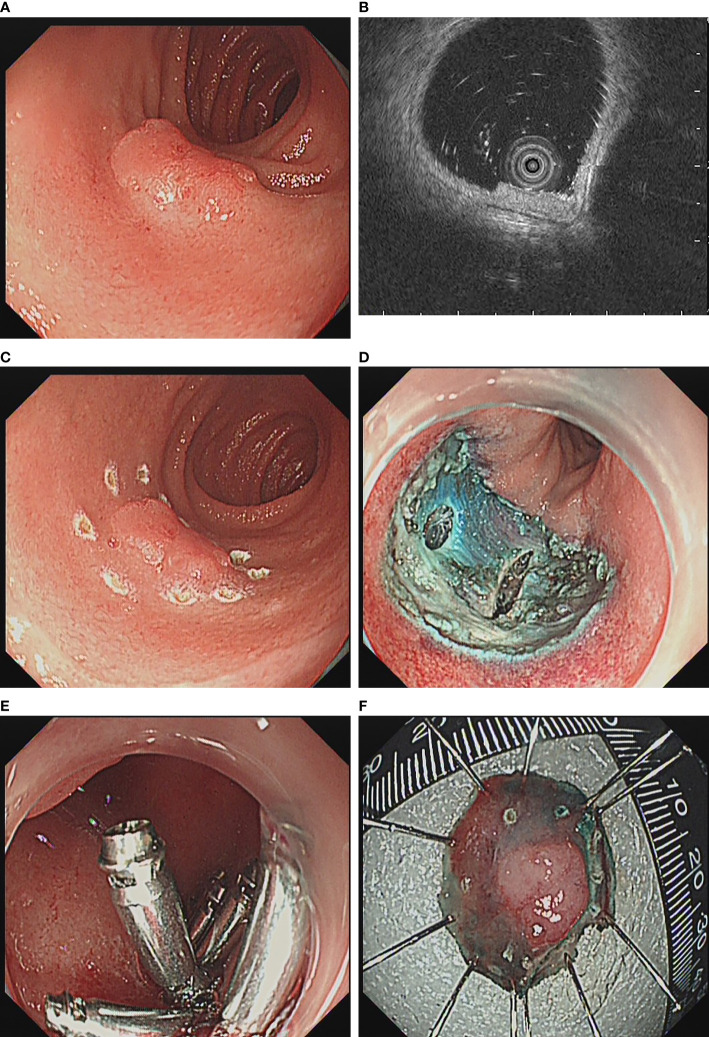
ESD for duodenal bulb lesions. (A) Lesion at the duodenal bulb; (B) Endoscopic ultrasonography revealed that the lesion was confined to the mucosal layer; (C) Dual knife was used to mark around the lesion; (D) Wound after lesion resection; (E) Close the wound with a metal clip; (F) Lesion after resection.
